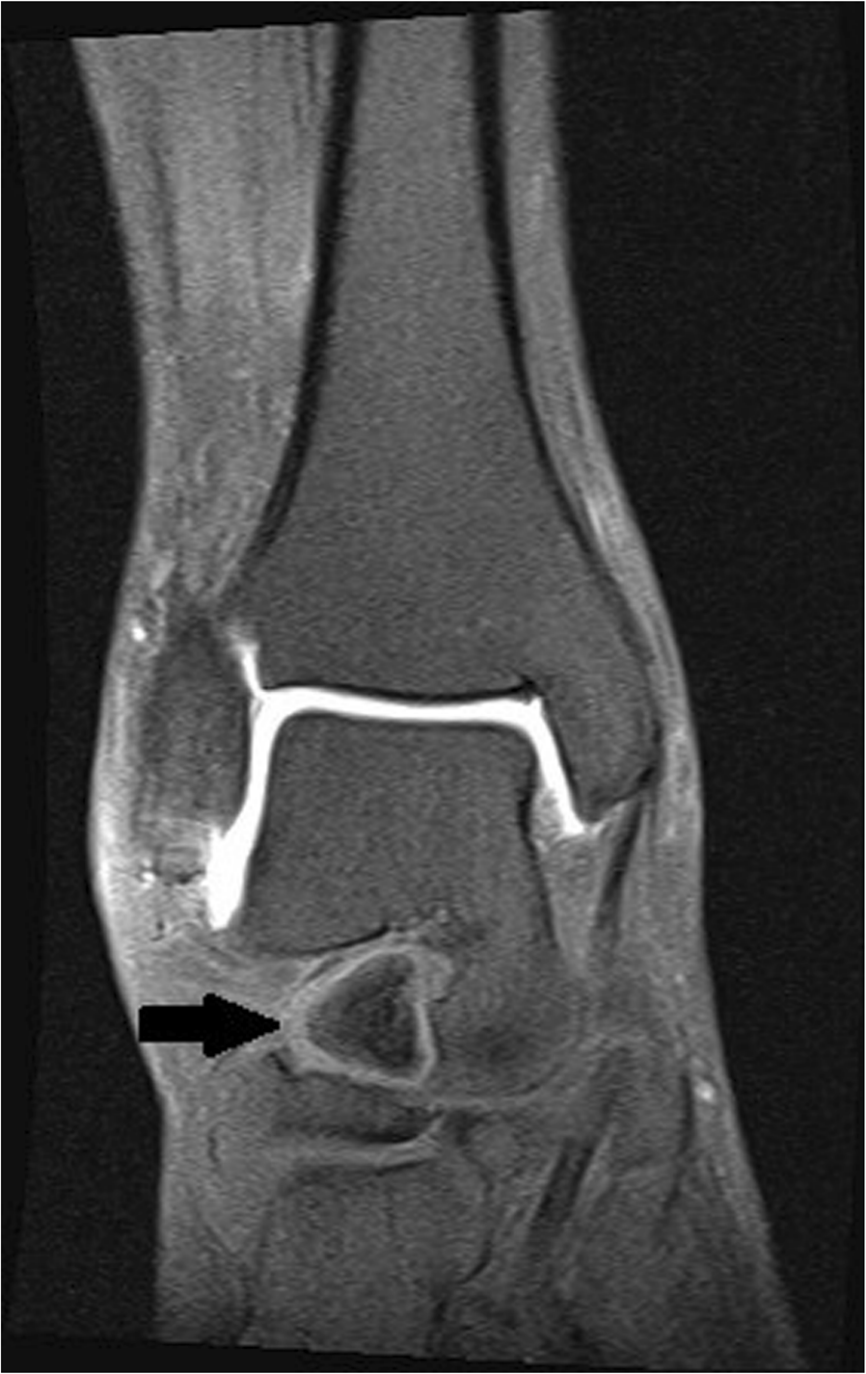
Frontal magnetic resonance imaging of the foot, T1-weighted image showing the calcaneus secundarius (arrow) preoperatively.
